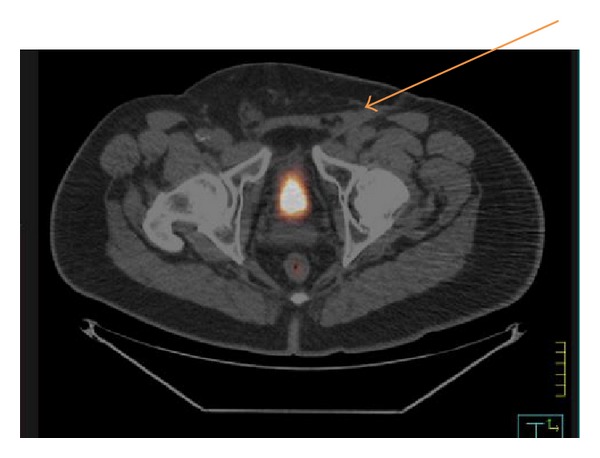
Most recent PET-CT revealing no evidence of disease uptake.
